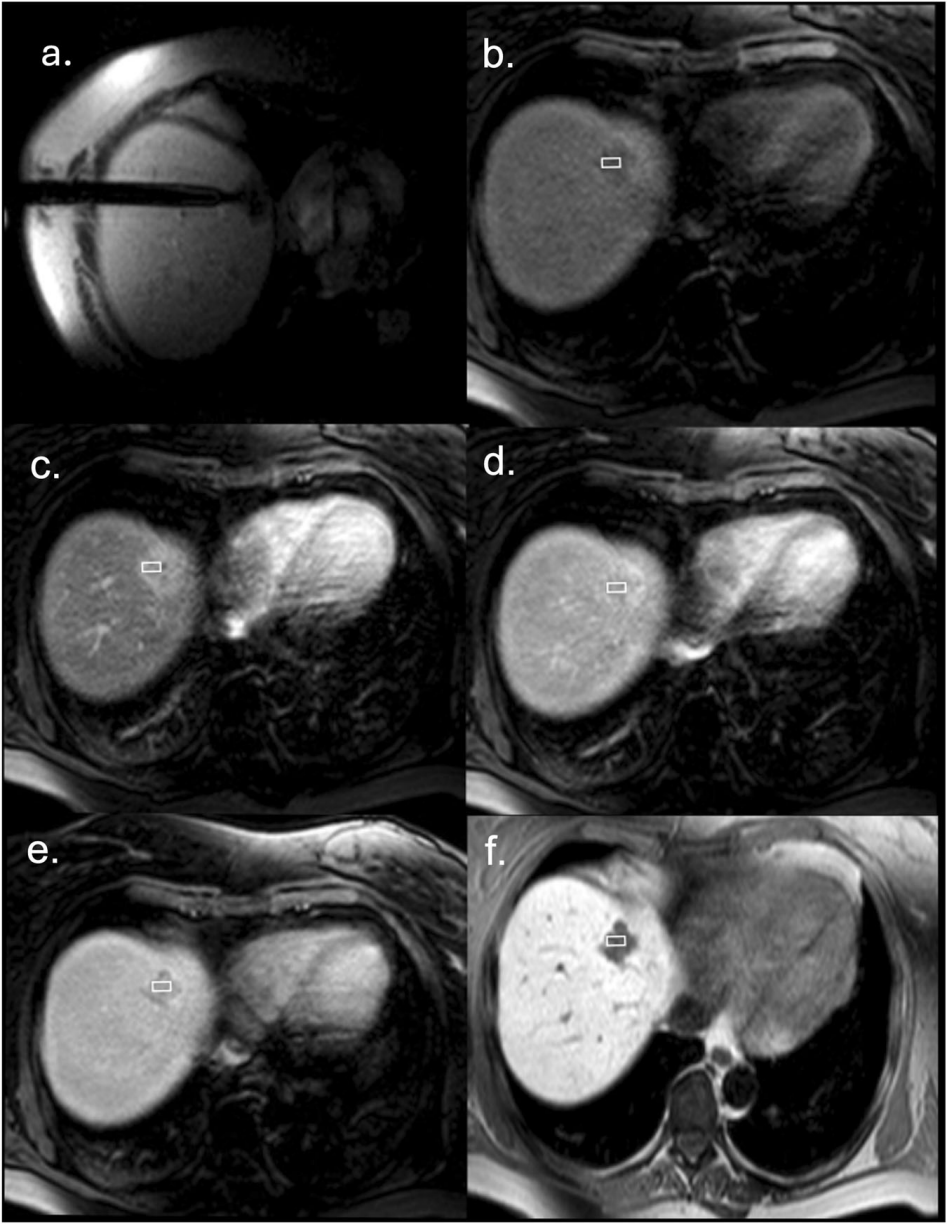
MRI findings of a BCLM. a MRI-guided biopsy image showing needle position. A rectangular region of interest is placed within the lesion in each phase according to the needle position on the biopsy image. b Non-contrast T1w MRI image. The SI at this stage is measured at 100. c Arterial phase T1w MRI image. The SI in this phase is 165, resulting in an RTE of 0.65. d Portal-venous phase T1w image. The SI in this phase is 130, with an RTE of 0.30. e Venous phase T1w image. The SI in this phase is 125, corresponding to an RTE of 0.25. f Hepatobiliary phase T1w image. In this phase, the SI drops to 40, resulting in a negative RTE of −0.60
